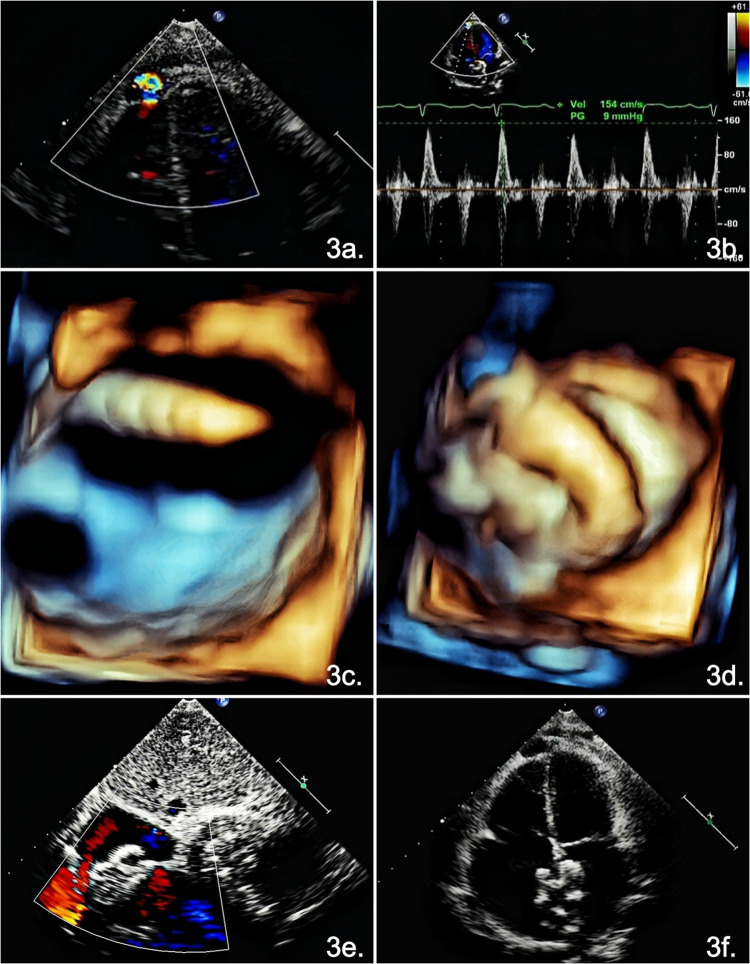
TTE images following pericardiectomy. (a) On the first postoperative day, TTE shows communication between the right ventricle and the apical outpouching. (b) Spectral Doppler at the neck showing a bidirectional flow signal. (c) Three-dimensional TTE showing two defects in the atrial septum, with the delivery sheath advanced through a large defect. (d) Three-dimensional TTE confirming complete seating of the double-disc occluder. (e) TTE demonstrating a well-positioned occluder without a residual shunt on the eighth postoperative day. (3f) Apical four-chamber view showing a persistent diverticulum.
